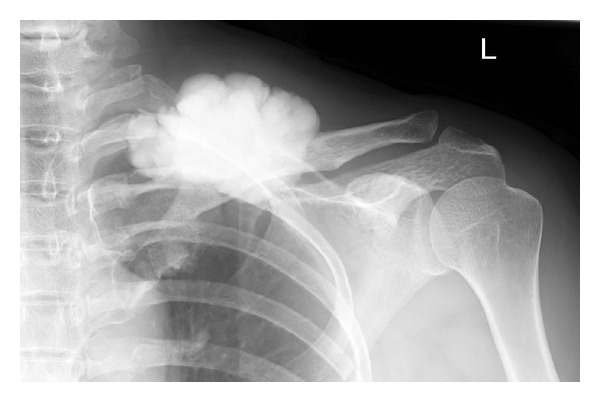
Radiographs reveal a well-defined, lobed mass in the midportion of the clavicle.
